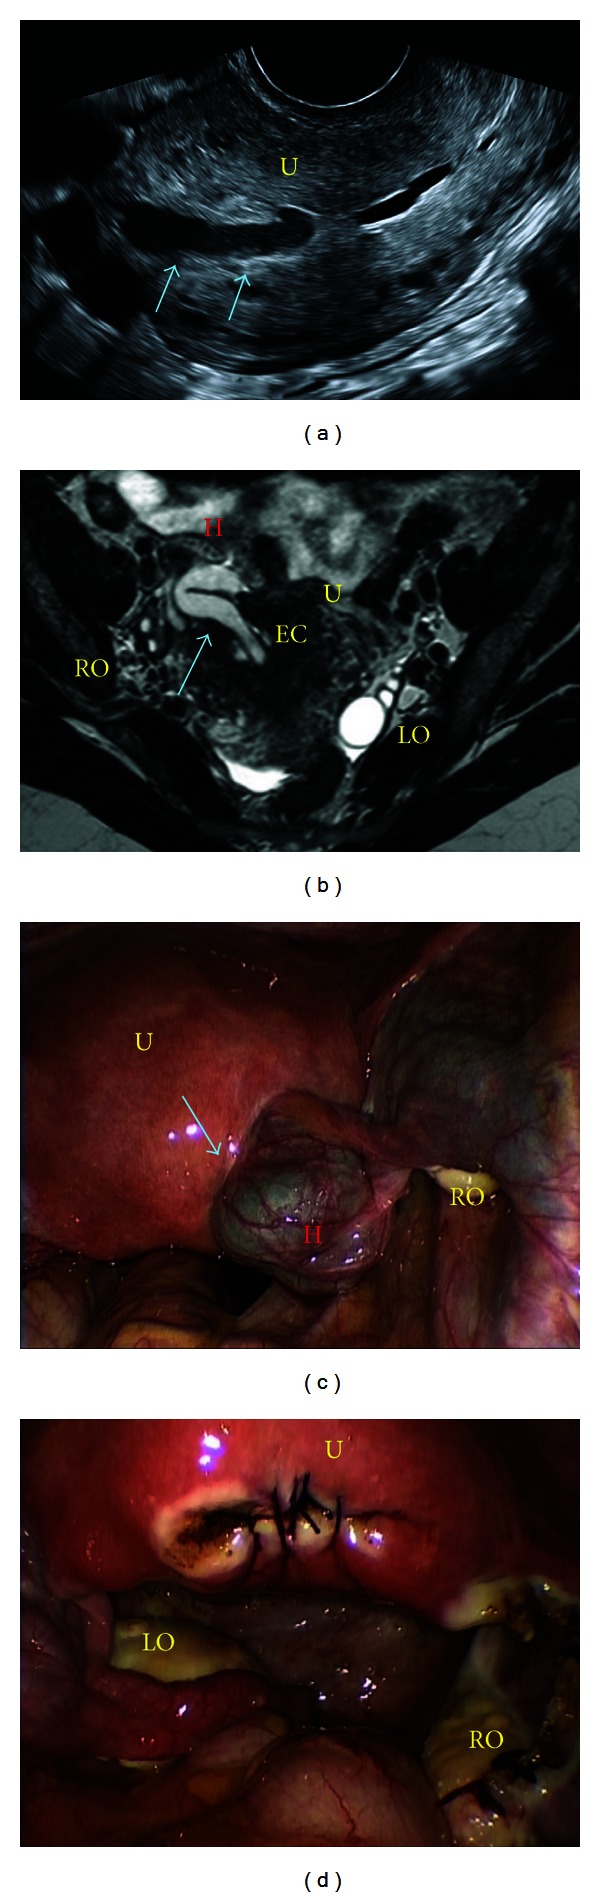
(a) Transvaginal ultrasound showing a hypoechoic structure (blue arrows) within the uterus (U). (b) Pelvic MRI demonstrating a herniation (blue arrow) of the right hydrosalpinx (H) through the uterine wall (U) going up to the endometrial cavity (EC). Both the right (RO) and the left (LO) ovaries were normal. (c) Laparoscopic visualization of the pelvic cavity: the hydrosalpinx (H) is incarcerated (blue arrow) in the uterus (U), and the right ovary (RO) is normal. (d) Final aspect of the procedure: uterus (U), left ovary (LO), and right ovary (RO).
